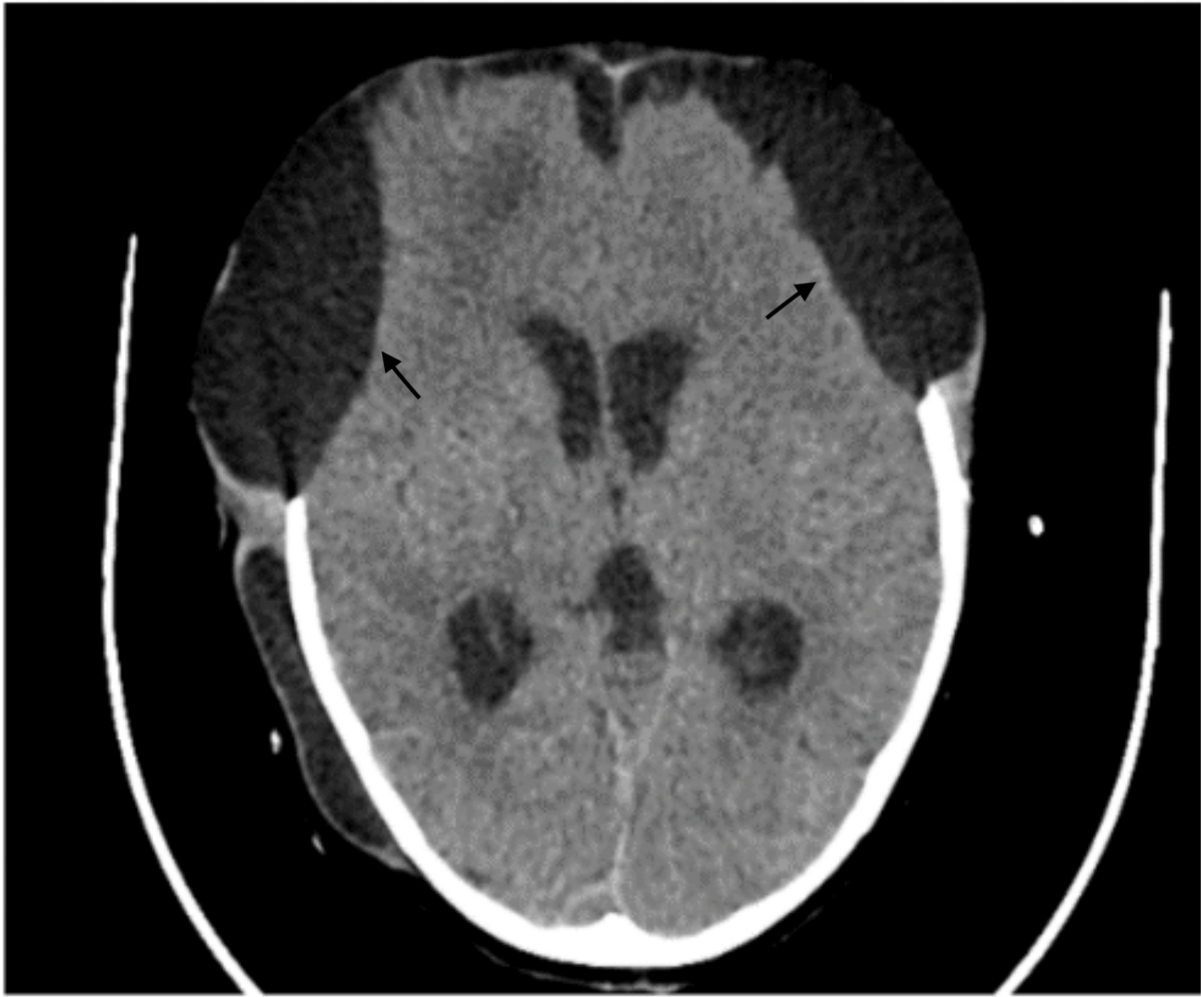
Postoperative MRI shows bifrontal decompressive craniectomy with lax duroplasty and insertion of an external ventricular drain (EVD).
